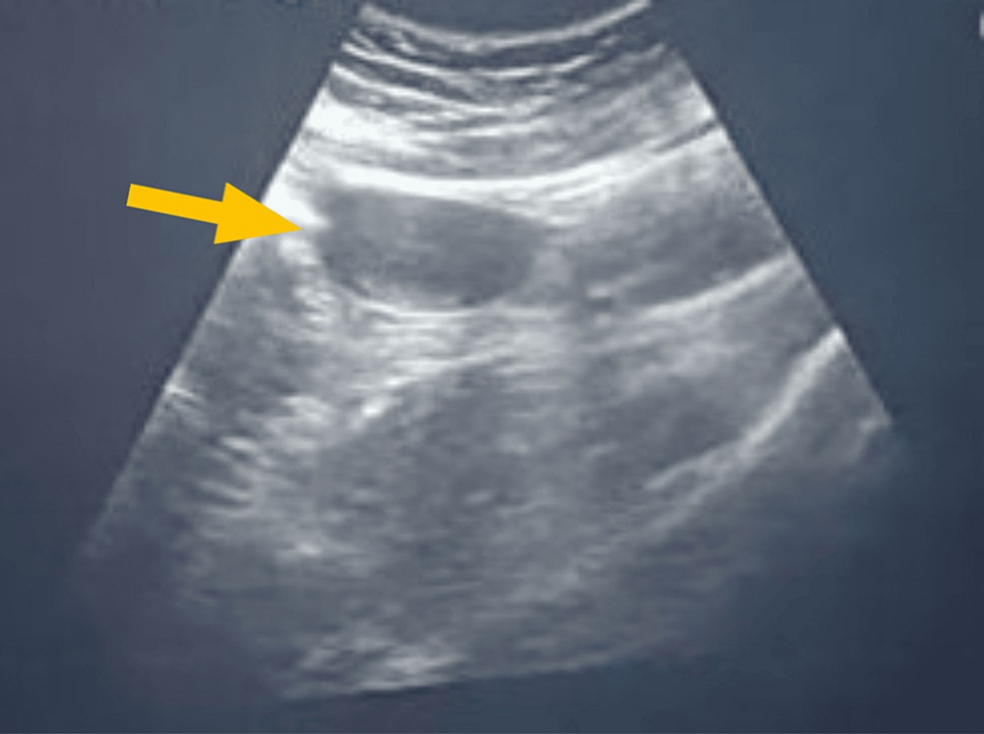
Sonography report of the patient depicting uterus with two horns
